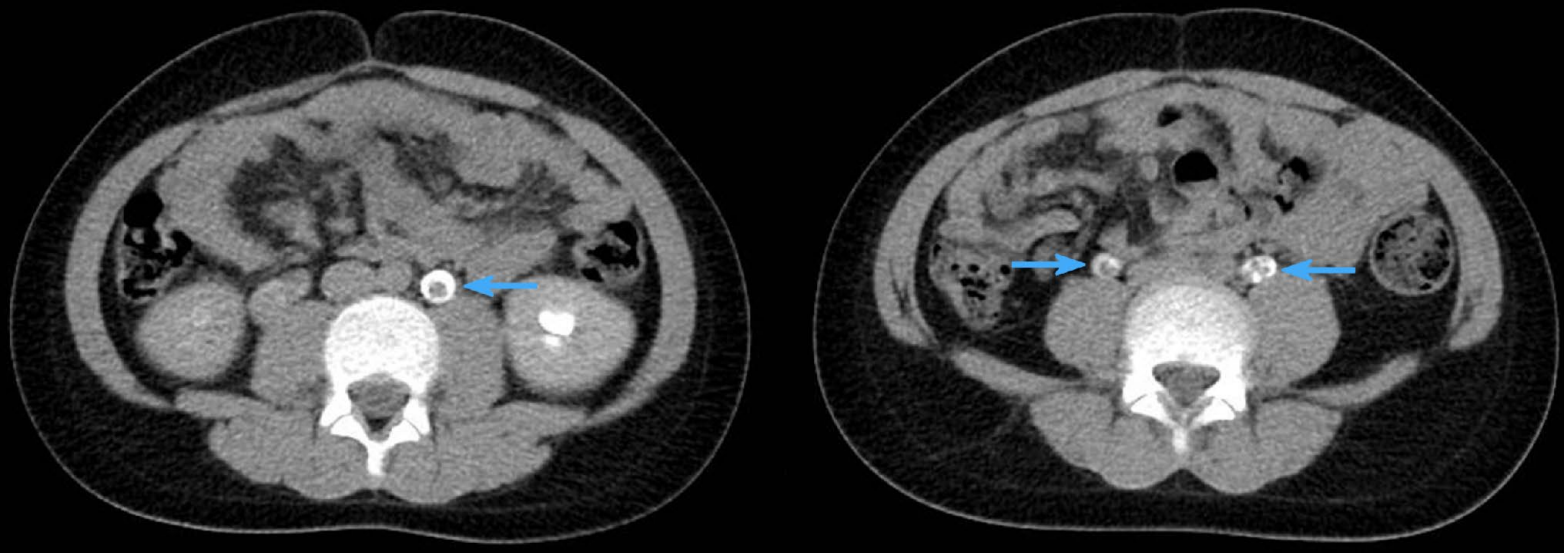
Delayed contrast enhanced CT scan of a 10-year-old boy with bilateral ureteral
